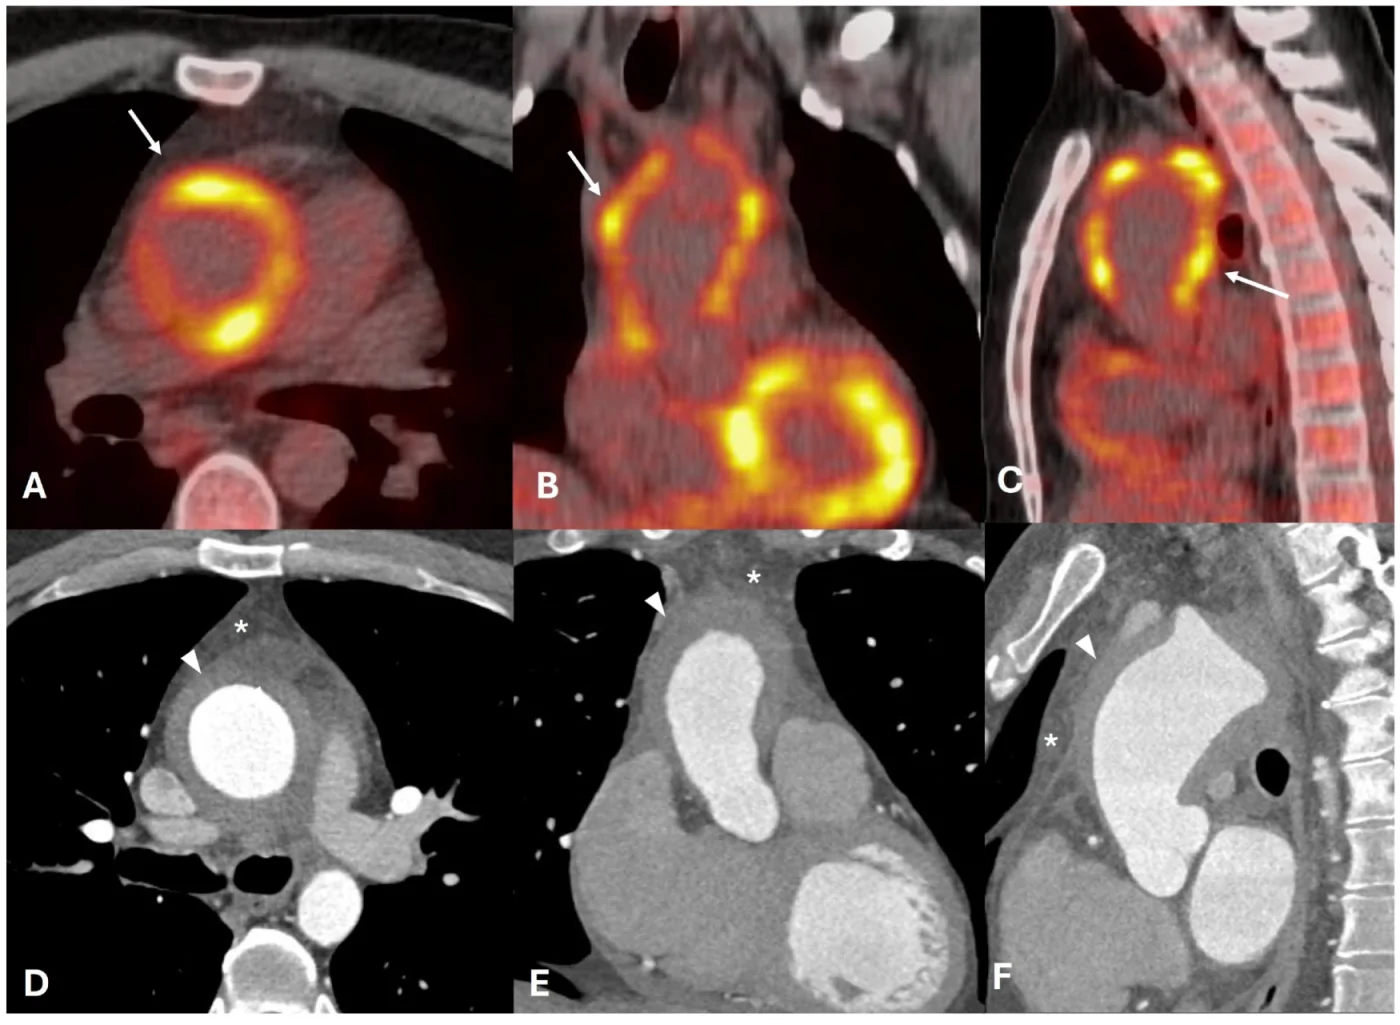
An example of known active relapsing polychondritis evaluated for chest pain. Axial (A), coronal (B) and sagittal (C) fused images of F-18 FDG PET/CT showing moderate intensity heterogeneous mural tracer activity throughout the ascending aorta involving the aortic root consistent with active aortitis (arrows). Axial (D), coronal (E) and sagittal (F) images of chest CTA showing concentric mural thickening to the ascending aorta (arrowheads) with surrounding infiltration to the mediastinal fat (*) corresponding to areas of abnormal tracer activity seen on PET/CT.
